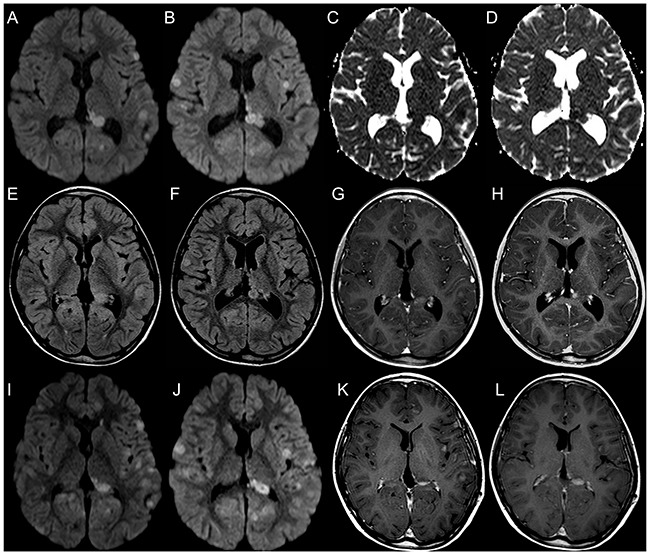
Brain MRI findings in a 12-year-old girl with multiple focal distant medulloblastoma relapsing lesions better depicted by DWI DWI images (A, B) and corresponding ADC maps (C, D) reveal multiple leptomeningeal and ependymal focal nodular lesions with decreased diffusivity. FLAIR (E, F) images show a lower number of lesions, and only few of them demonstrate contrast enhancement (G, H). On follow-up MRI, DWI (I, J) and post-contrast T1-weighted images (K, L) demonstrate increased number and volume of focal lesions, confirming multifocal disease relapse.
